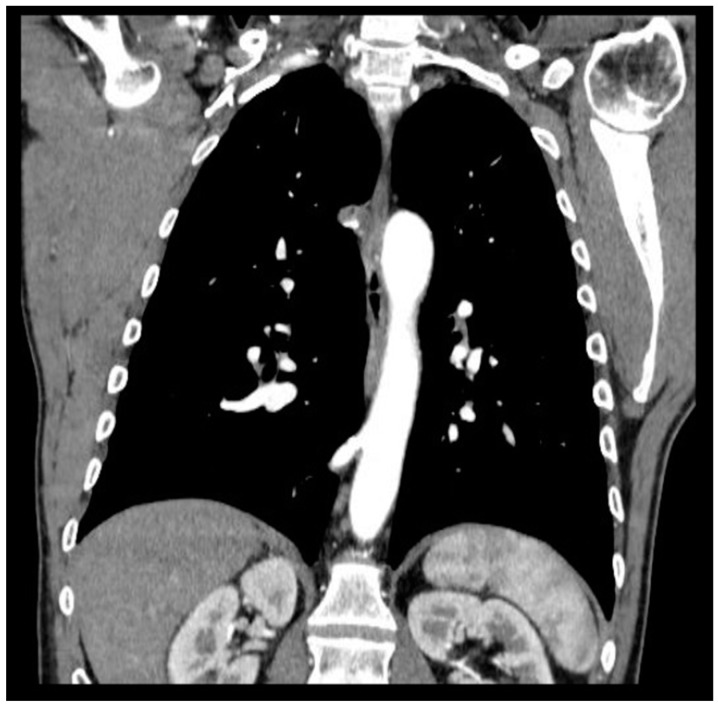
CT evidence of systemic arterial vascularization from descending thoracic aorta.
